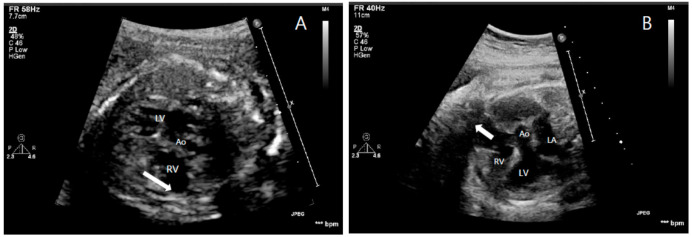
Fetal echocardiogram at 23 + 3 weeks of gestation (A) shows dysplastic RV cavity and focal thinning of RV free wall myocardium (white arrow) and at 35 + 1 weeks of gestation (B) shows aneurysmal dilatation of the RV (white arrow). RV, right ventricle; LV, left ventricle; LA, left atrium; Ao, aorta.
